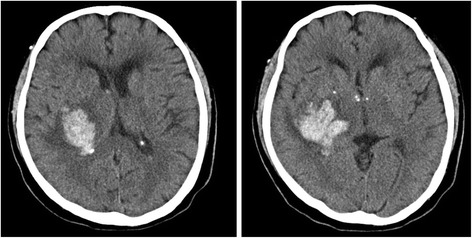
Axial brain computed tomography of the patient. The hemorrhage at the right basal ganglia extended medially to the thalamus and laterally to the external capsule
